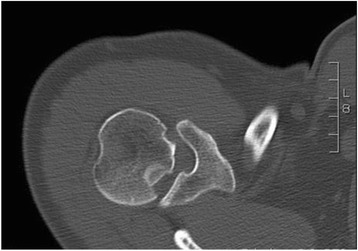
CT scan after posterior shoulder dislocation
